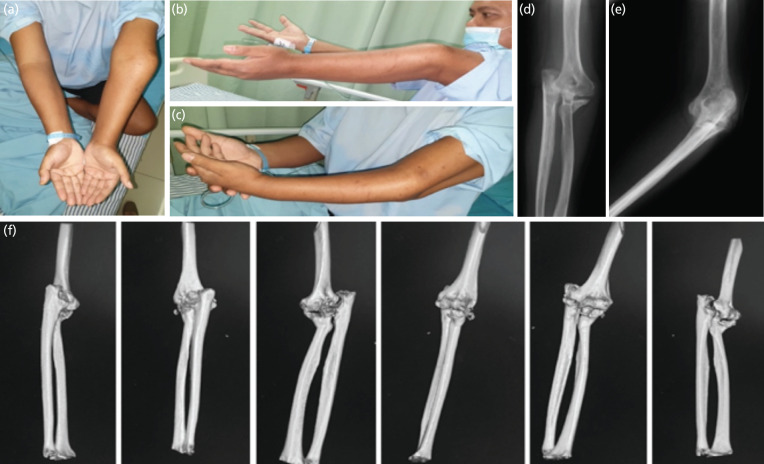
(a, b, c) Pre-operative clinical pictures, (d, e) Plain radiographs, and (f) CT Scan result of the patient.
